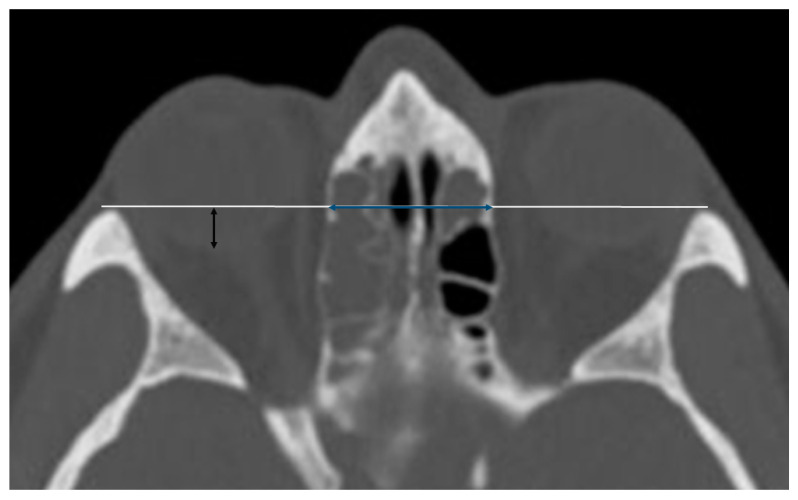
Sinus computed tomography scan, axial plane. White line: horizontal line drawn from the medial tip of the lateral orbital rim to the contralateral orbital rim. Blue line: distance between the two laminae papyracea in the axial plane (IOD_Axial_). Black line: represents the antero-posterior position of the right globe (PGP).
